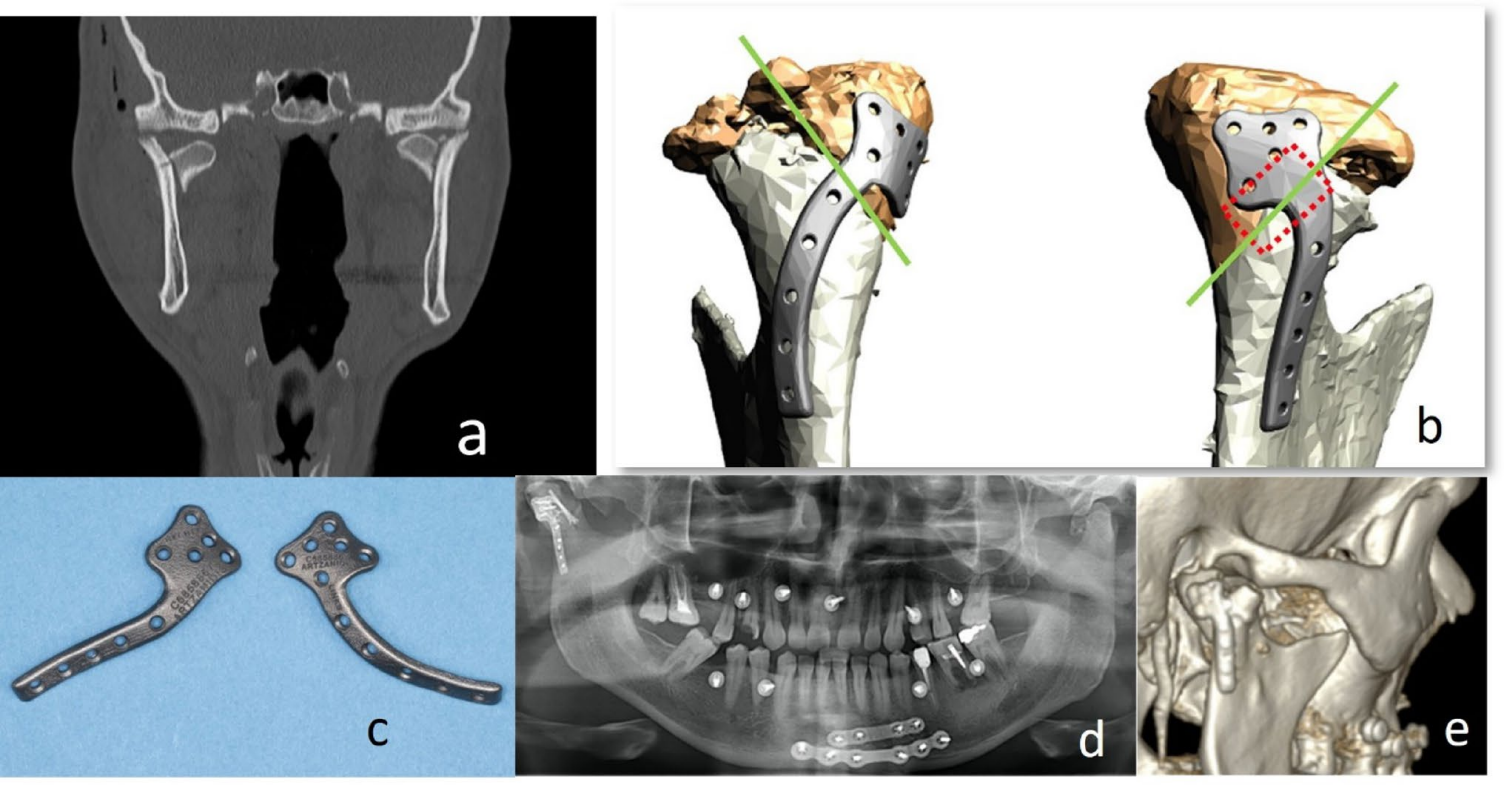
Case 1: Preoperative CT-Scan (coronal plane) – bilateral mandibular CHF: right side – AO type-p without fragmentation and lost vertical apposition; left side – AO type-p with minor fragmentation and lost vertical apposition (a). VSP and CAD dorsolateral views of both CHF – green lines indicate the fracture plane; right CHF with fracture line widely covered by the rhomboid PSD shield (red rectangle) (b). CAD/CAM PSDs (KLS Martin, Tuttlingen, Germany) (c). Postoperative Imaging – Panorex (d); 3D CT-Scan – dorsolateral view (e)
